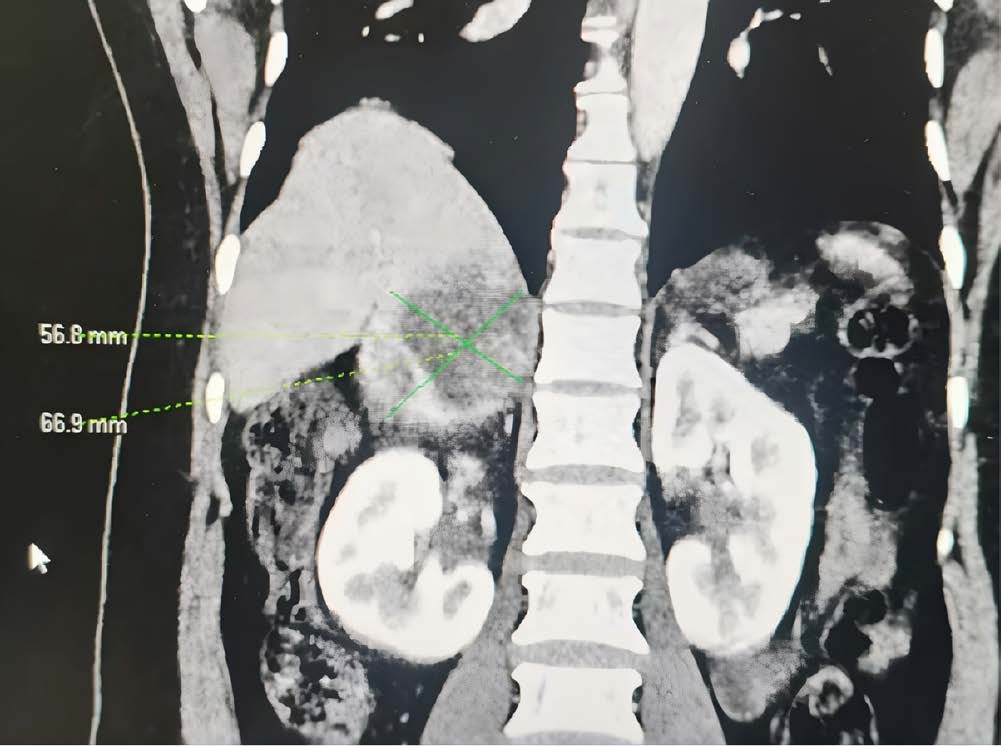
Contrast-enhanced CT scan showing a right adrenal mass (yellow arrow). CT = computed tomography.
